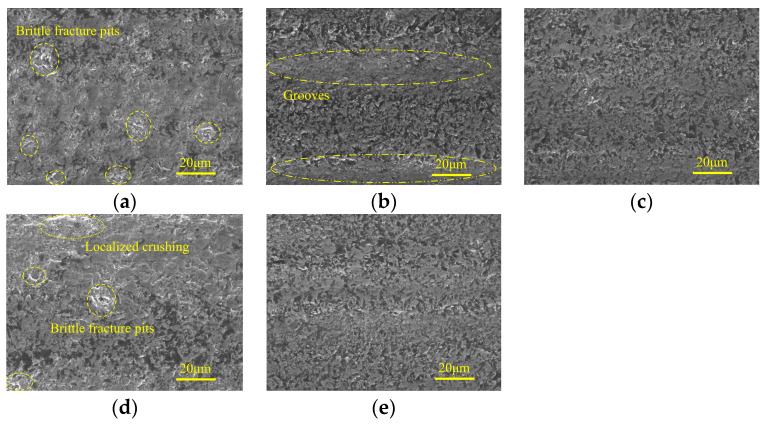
Micro-morphologies under different ultrasonic amplitudes in rough grinding stage: (a) $T_{A}$: 0 μm $W_{A}$: 4 μm; (b) $T_{A}$: 4 μm $W_{A}$: 4 μm; (c) $T_{A}$: 8 μm $W_{A}$: 4 μm; (d) $W_{A}$: 0 μm $T_{A}$: 4 μm; and (e) $W_{A}$: 8 μm $T_{A}$: 4 μm.
